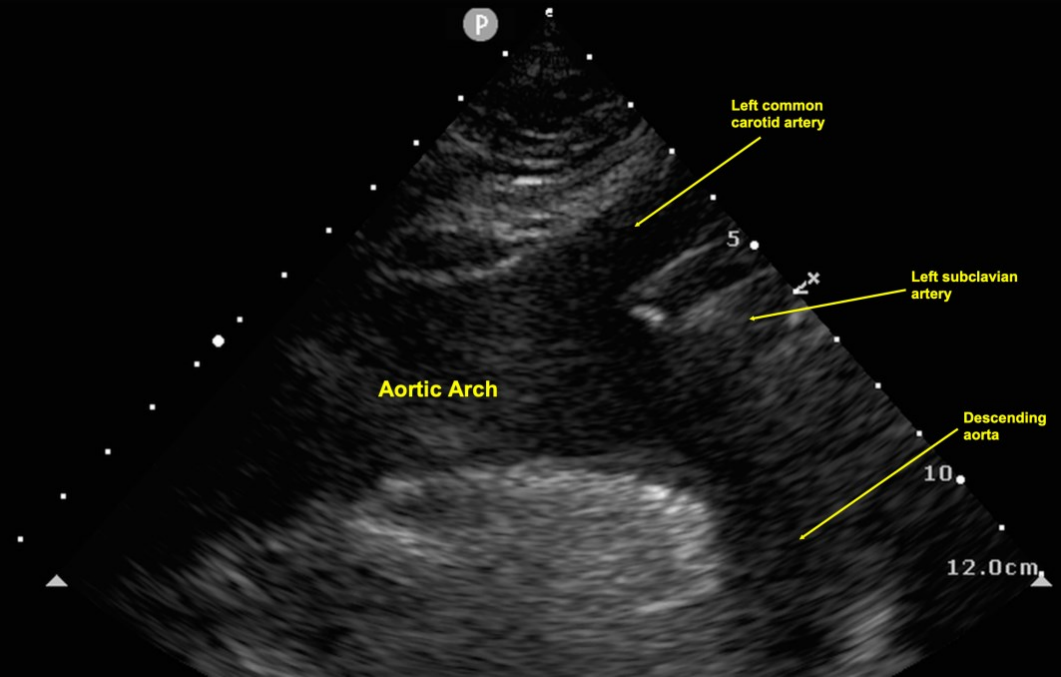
Normal aortic arch anatomy as seen on a suprasternal aortic notch POCUS. Note, this is the emergency medicine convention for probe and screen marker, not the echocardiography convention.
